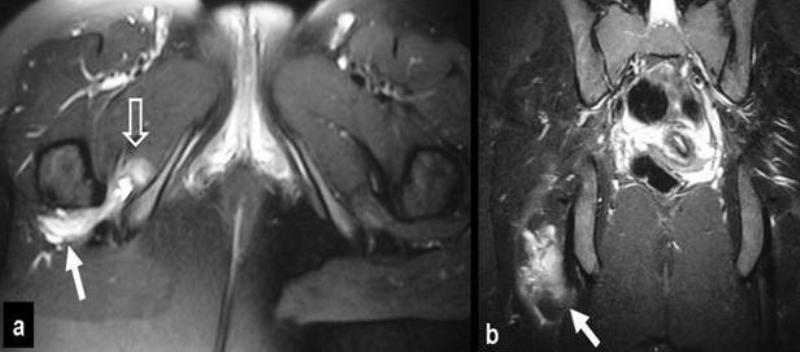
MRI performed a few days after injury. (a) The transverse fat suppressed proton density turbo spin echo (TSE) and (b) the coronal short tau inversion recovery (STIR) images, show the hematoma formation in the quadratus femoris muscle (arrows) extending to the obturator internus muscle (open arrows).
